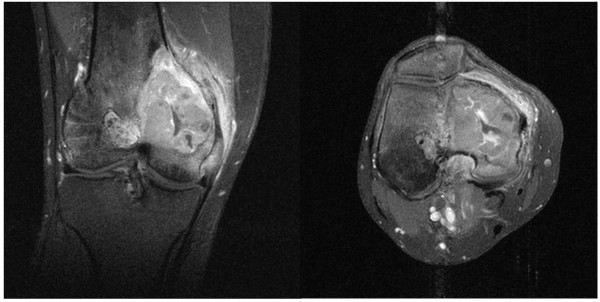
Coronary (a) and transversally (b) T1-weighted MRI sequence shows a bone tumor spreading across the medial cortical bone and infiltrating into the vastus medialis muscle and the medial retinaculum. The graft and the femoral drill tunnel are not infiltrated by the MFH.
